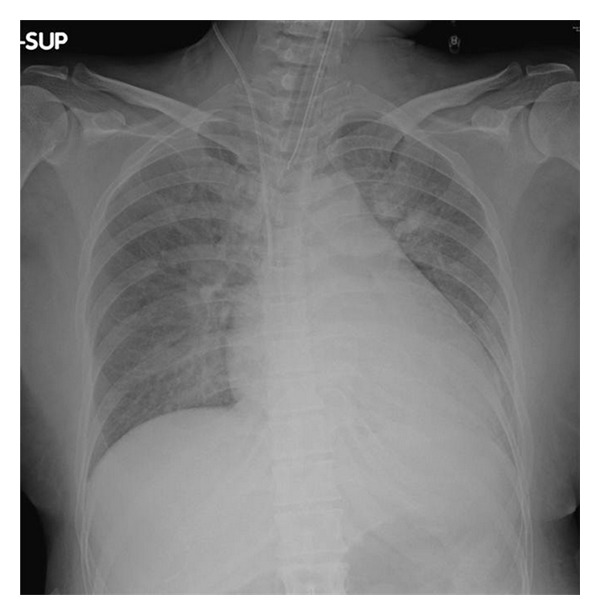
Chest X-ray on admission showing bilateral pulmonary infiltration and cardiac dilatation.
